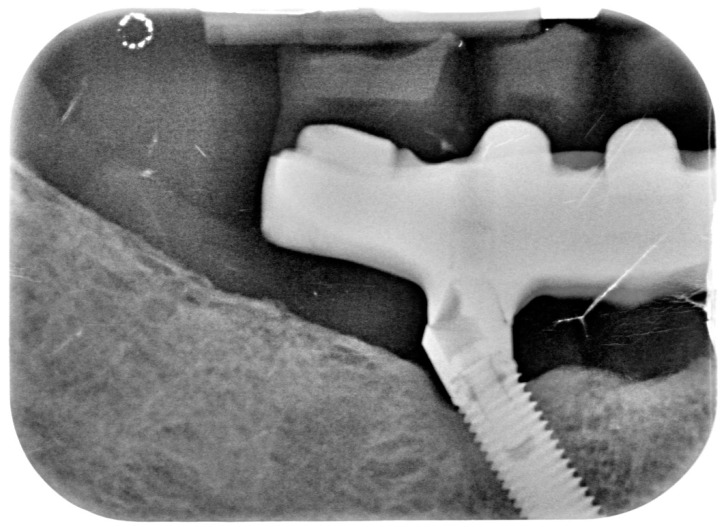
X-ray of 4.5 dental implant in patient before treatment.
